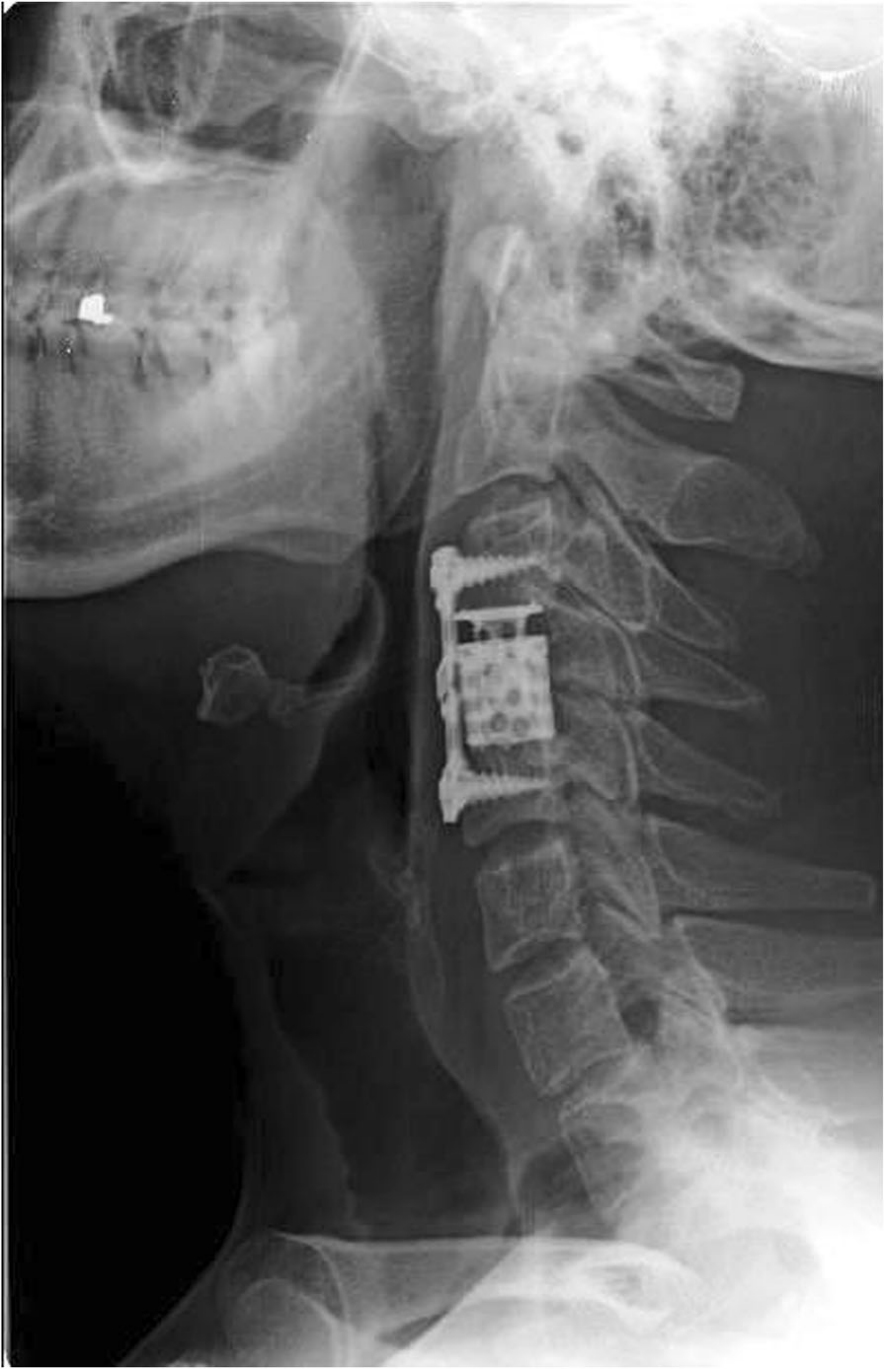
No recurrence was seen on the direct X-ray taken in the postoperative third year
